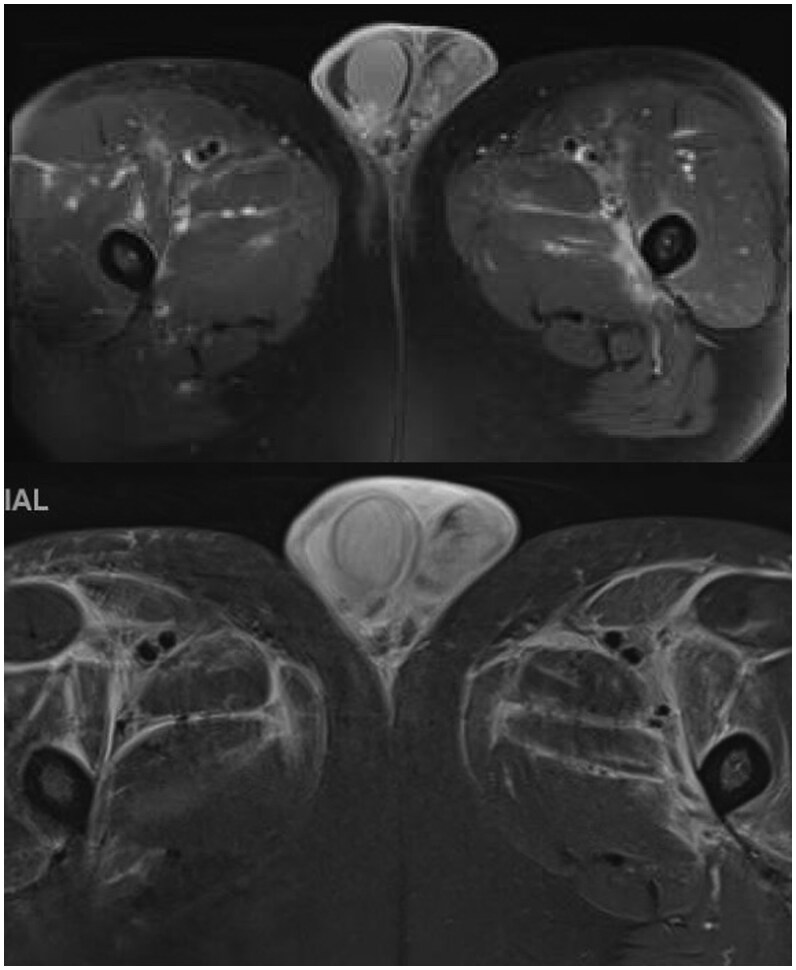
MRI abdomen and pelvis with and without contrast. Scrotal wall edema and small right hydrocele observed on T1 with fat suppression and gadolinium (upper) and STIR (lower). There is marked diffuse superficial, deep edema and muscular enhancement in the bilateral proximal thighs and pelvis, concerning for systemic vascular inflammatory process, myositis, or cellulitis.
